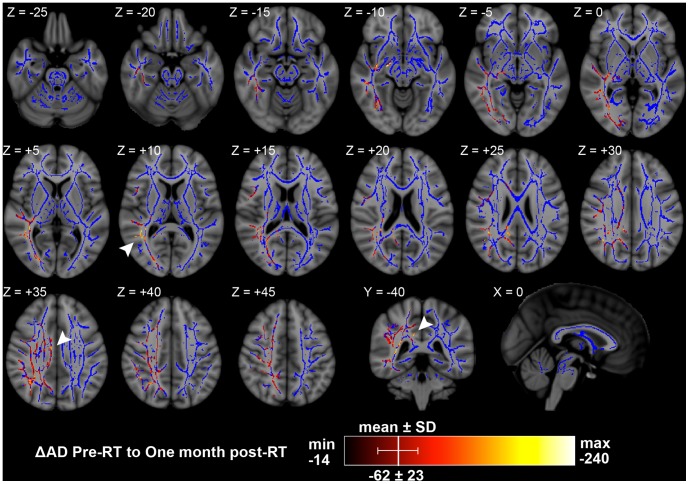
Significant changes in AD from pre-RT to one month post-RT. Arrowheads: Z = +10, right superior longitudinal fasciculus; Z = +35, right superior cingulum; Y = −40, right superior cingulum. Significant results per color chart, blue is TBSS skeleton without significant results. Depicted on MNI ICBM152 standard brain T1-weighted image [34]. Units are µm^2^/s.
